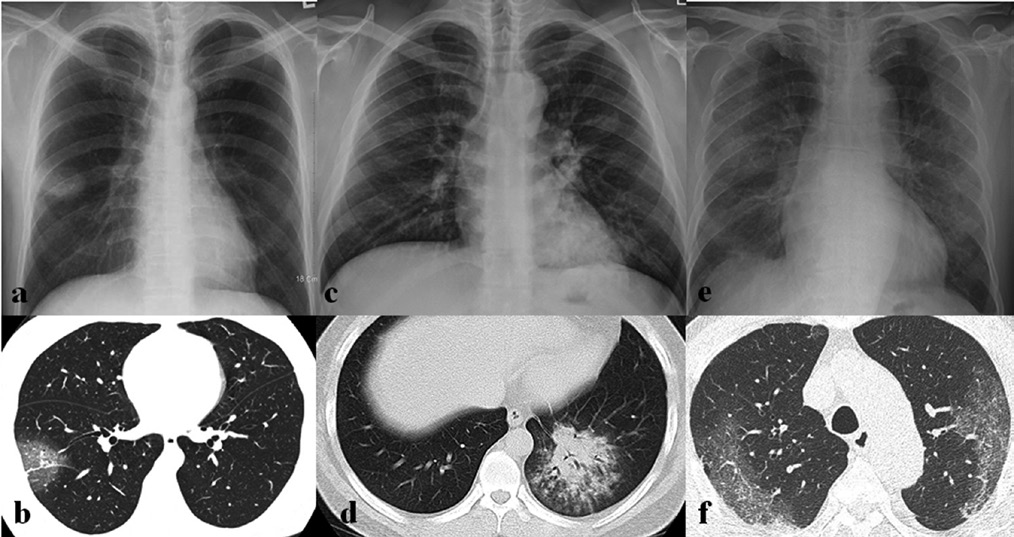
Initial imaging presentation of coronavirus infection. (a) Initial chest radiograph (CXR) obtained from a 52-year-old male patient with SARS-CoV infection shows a focal nodular opacity in the peripheral area of right middle lung zone. (b) Initial CT scan obtained from a 47-year-old female patient with SARS-CoV infection shows focal area of ground-glass opacity (GGO) with crazy paving appearance in the peripheral portion of right lower lobe. (c, d) Initial CXR and CT scan obtained from a 36-year-old male patient with MERS-CoV infection show multifocal patchy consolidation in left middle to lower lung zones on CXR (c) and focal airspace consolidation with air-bronchogram, ill-defined nodules, and peripheral GGOs on CT scan (d). (e, f) Initial CXR and CT scan obtained from a 73-year-old male patient with SARS-CoV-2 infection show bilateral multifocal GGOs on CXR (e) and patchy areas of GGO with crazy paving appearance with peripheral predominance on CT scan (f).
